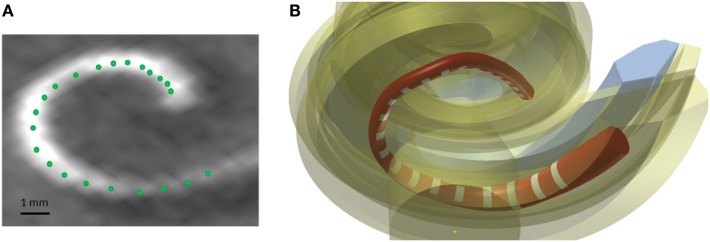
(A) Postoperative CBCT scan with the electrode positions. (B) CAD model of a Nucleus contour advance electrode array. The red color represents the silicon electrode carrier, the white color represents the electrode contacts, and the yellow color represents the different structures of the cochlea.
